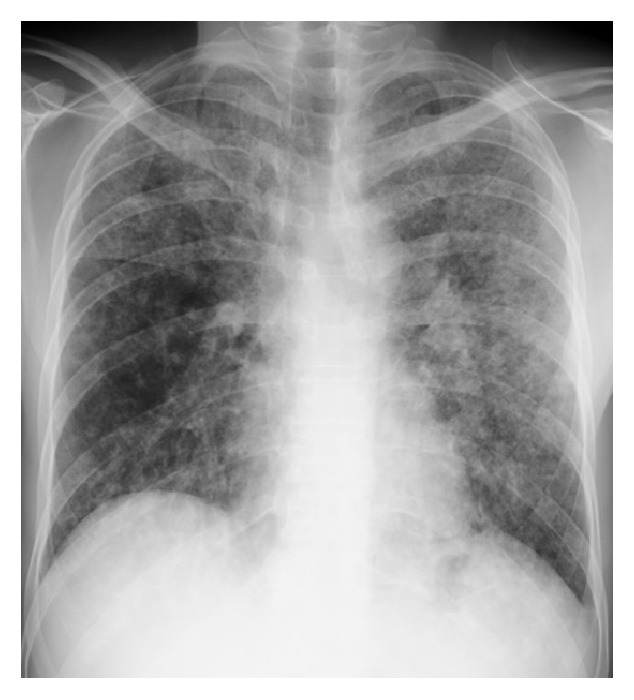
A chest radiograph obtained on the day of admission showed bilateral diffuse alveolo-interstitial infiltration, particularly in the left lung.
